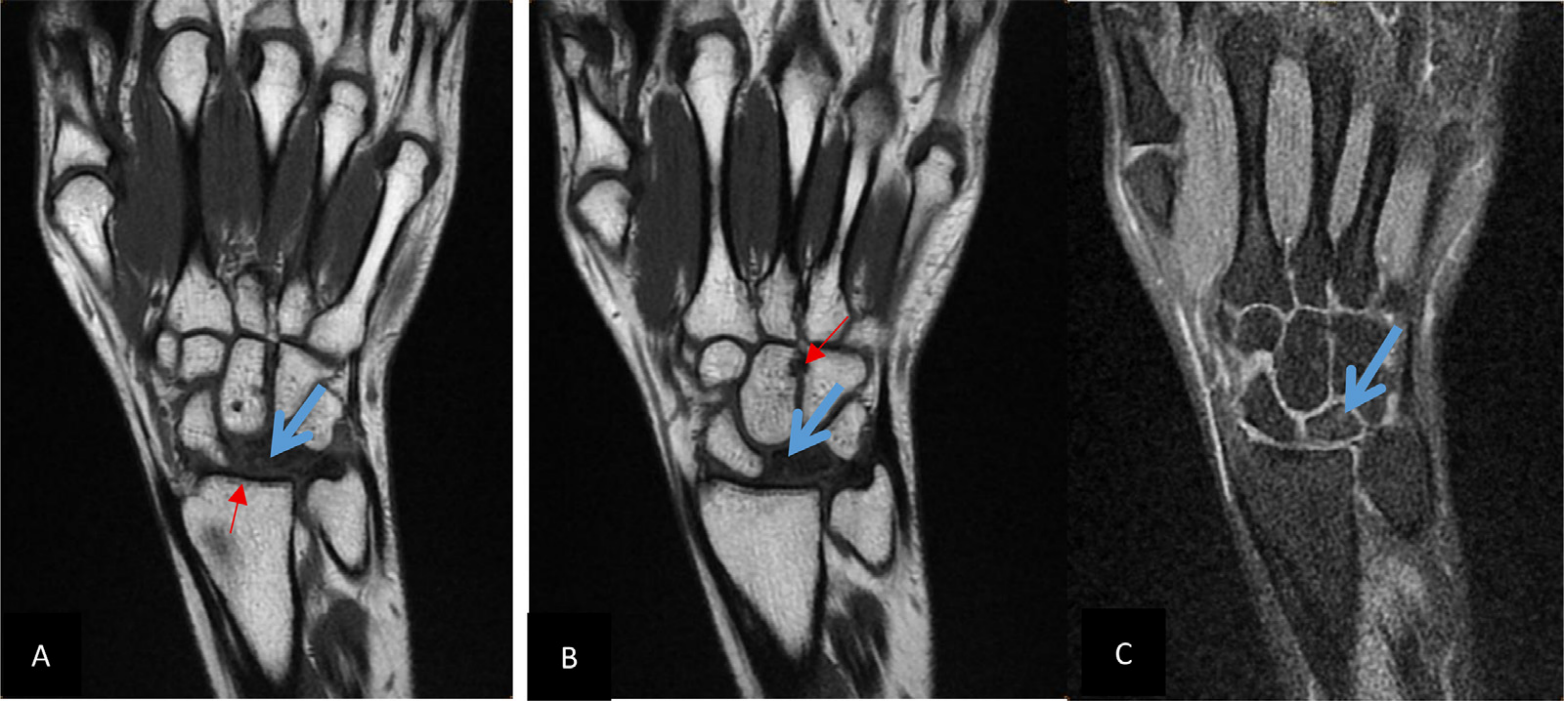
MRI of the right hand T1-weighted coronal sequence (A and B), and T1-weighted coronal sequence with fat suppression and gadolinium injection (C) demonstrates a hypointensity of the lunate bone and collapse (blue arrow) with osteoarthritic changes in radio lunate and mid-carpal joints (red arrow).
